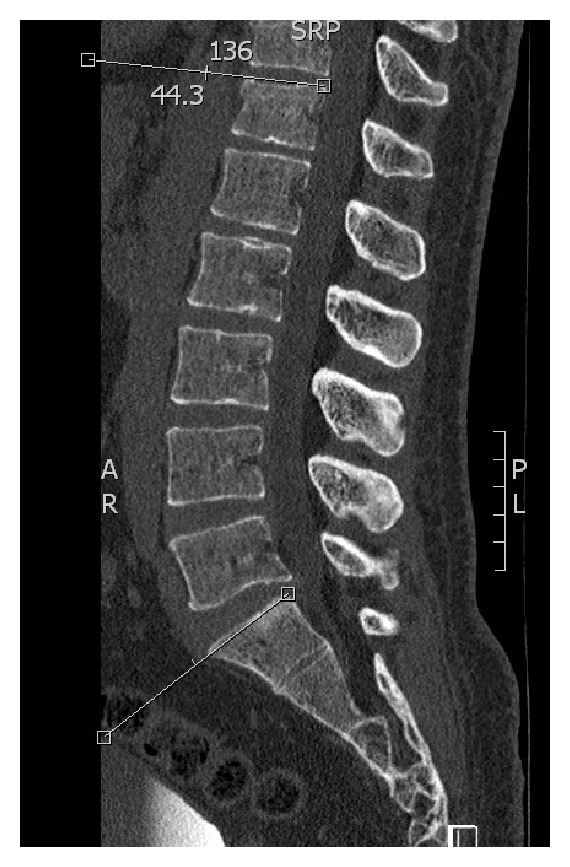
Functional Cobb angle measurement by CT.
